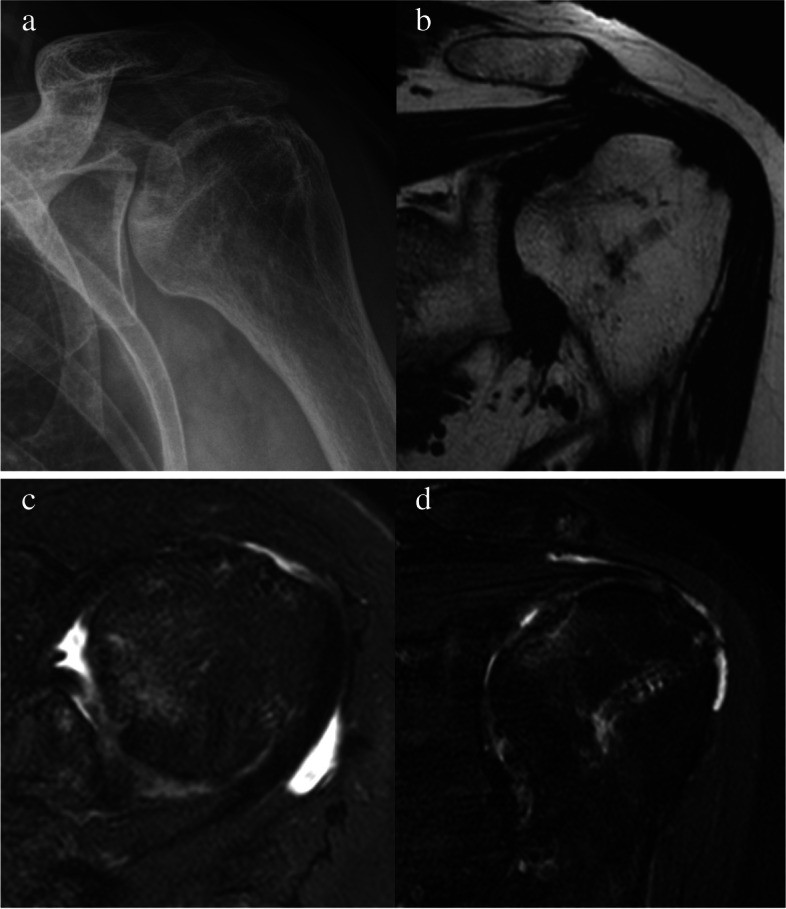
a, b, c Post-operative one-year follow-up plain radiography and MRI; osteonecrosis of the humeral head on the superomedial side can be seen in the T1-weighted coronal and T2-weighted axial view d in the T2-weighted coronal view, the repaired rotator cuff tendon shows insufficient thickness although discontinuity is not visible (Sugaya classification type III)
